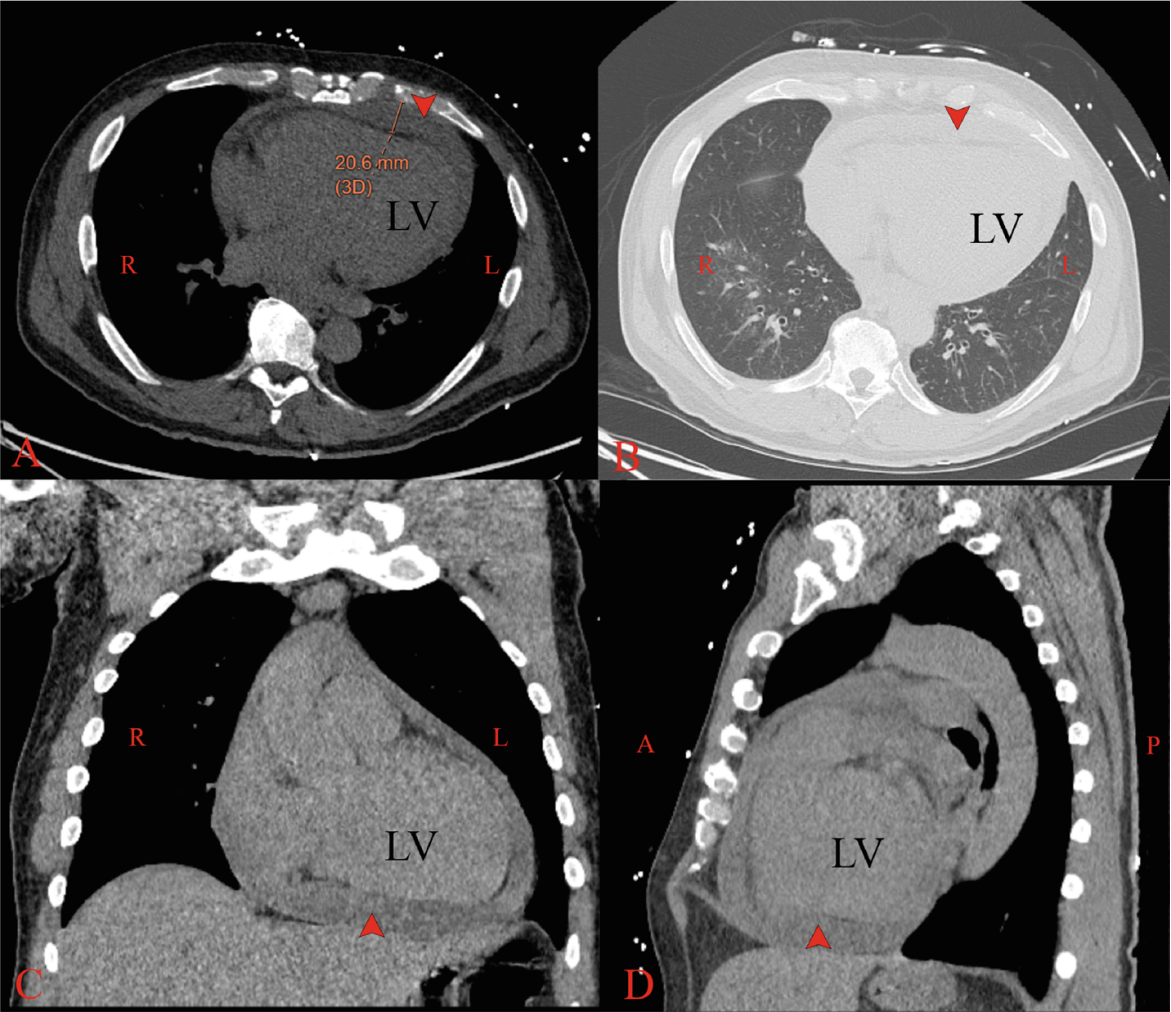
(A–D) Thoracic computed tomography showing pericardial effusion (red arrow). A: anterior, P: posterior, R: right, L: left, LV: left ventricle.
